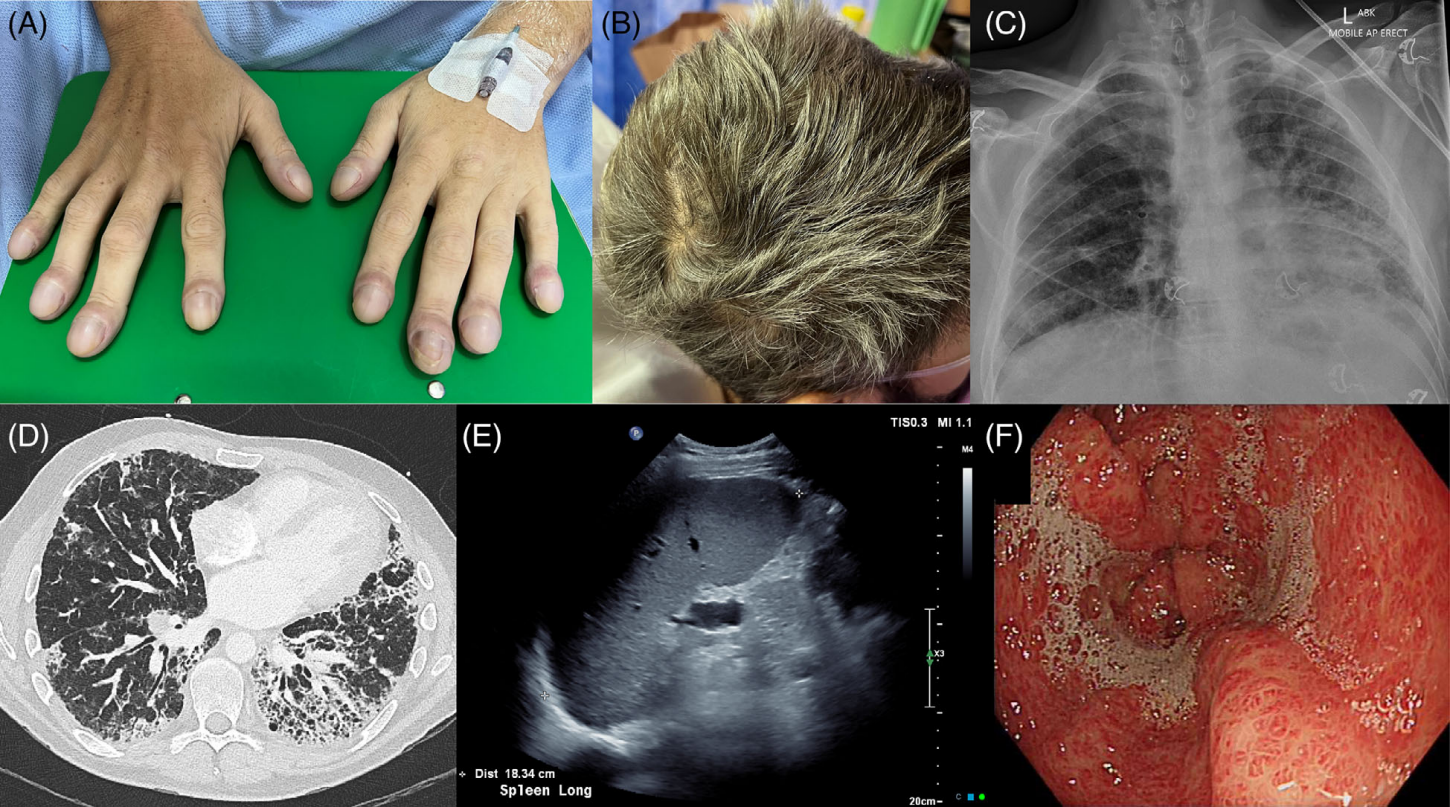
A 33‐year‐old man with pulmonary fibrosis and hepatopulmonary syndrome secondary to a telomere biology disorder. Clinical examination findings included (A) clubbing and (B) greying of hair, with (C) chest x‐ray demonstrating bilateral widespread patchy reticular opacities, more pronounced in the left lung. (D) High‐resolution computed tomography revealed a usual interstitial pneumonia pattern of pulmonary fibrosis. Cirrhosis with portal hypertension was confirmed by the presence of (E) splenomegaly on ultrasound and (F) portal hypertensive gastropathy identified during upper endoscopy.
